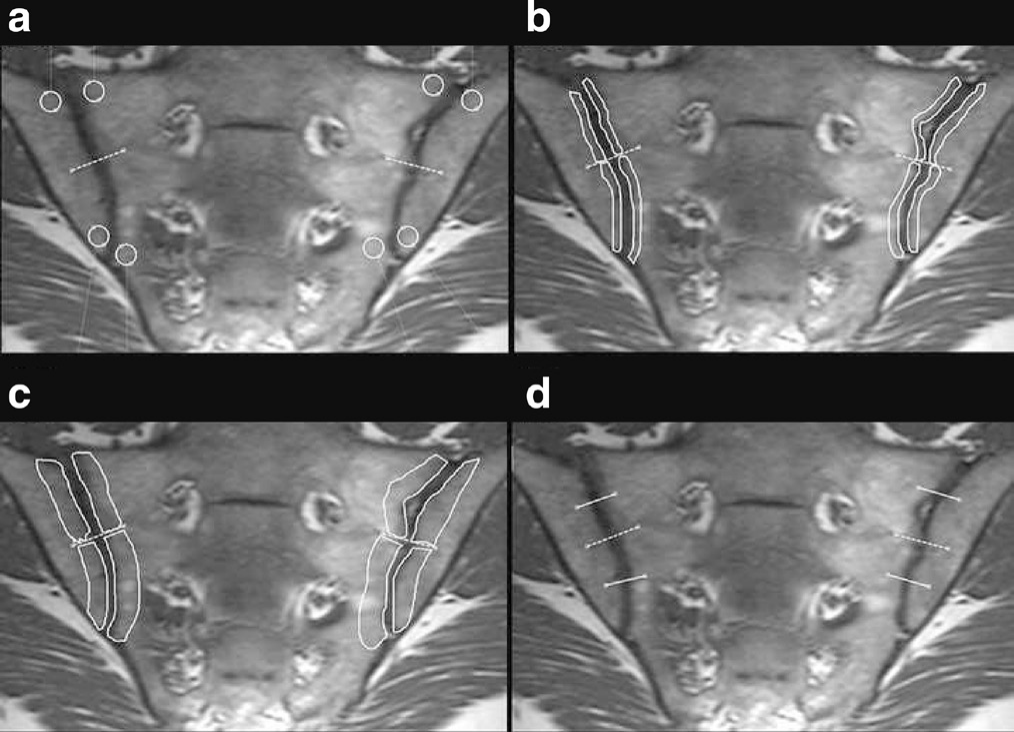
Semicoronal T1-weighted magnetic resonance image showing the four ROIs that were assessed: 40 mm^2^ circular (a); 5 mm anatomic band-shape (b); 10 mm anatomic band-shape (c); and 15 mm linear (d). ROIs, regions of interest.
